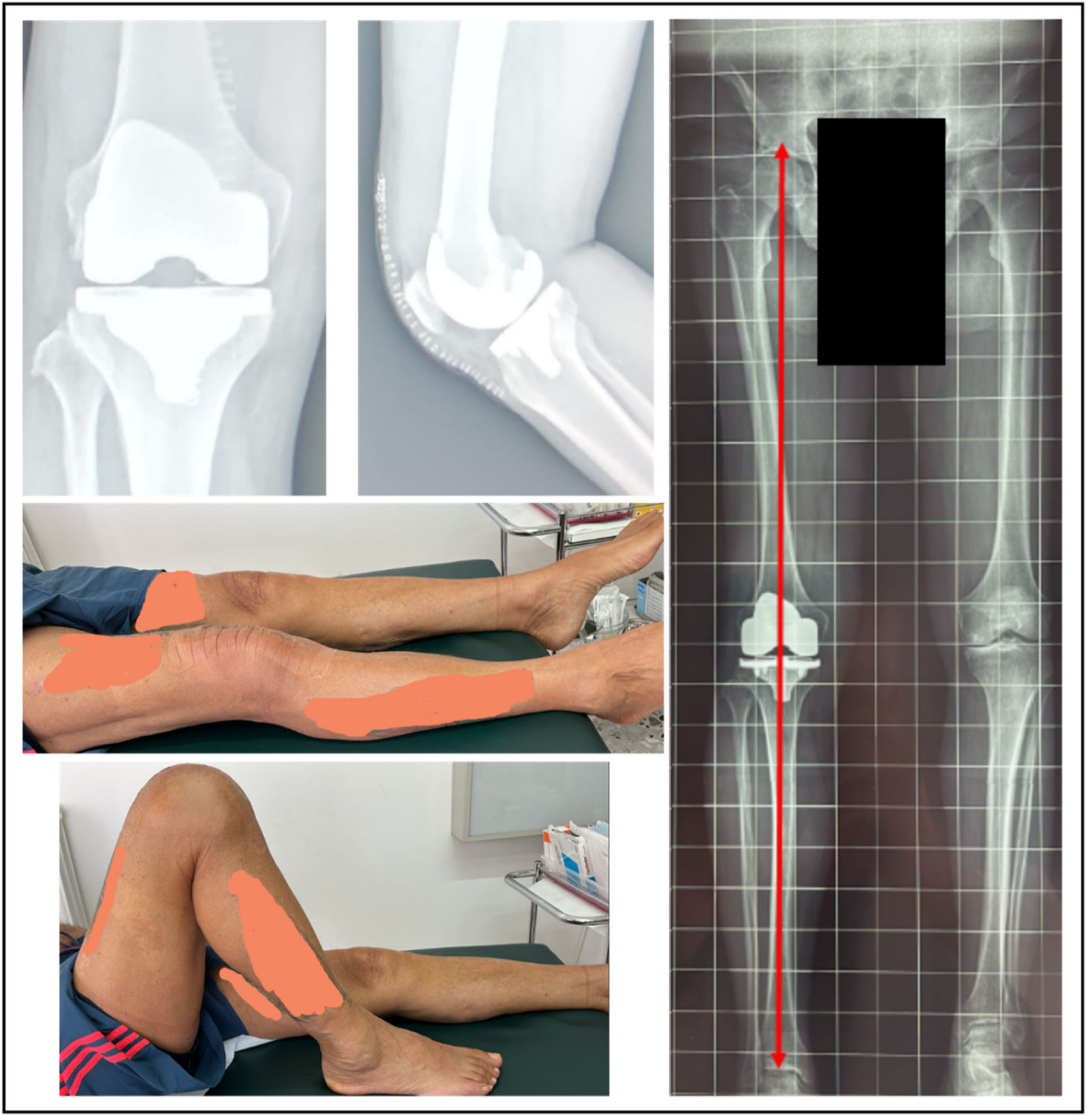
Post‐op radiographs and 3‐month post‐op photographs (*Stryker Triathlon*).
